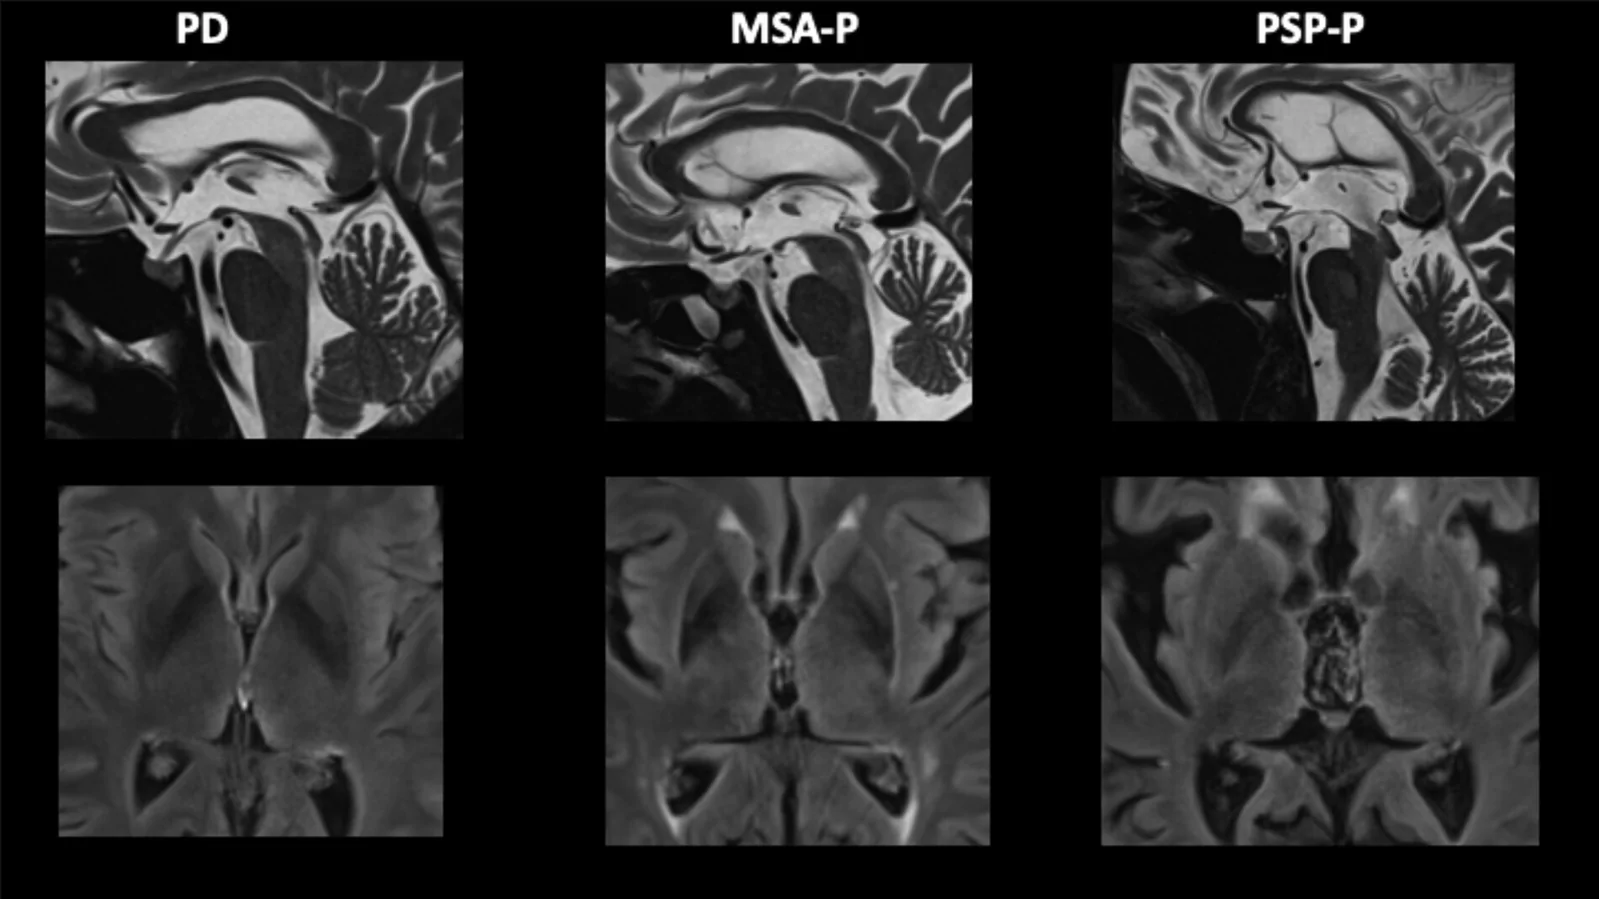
MRI features supporting the differential diagnosis of parkinsonian tremor syndromes. Conventional MRI is frequently normal in idiopathic Parkinson's disease (PD), whereas atypical parkinsonian syndromes may show characteristic structural abnormalities. In multiple system atrophy (MSA), these include putaminal atrophy and a hyperintense lateral putaminal rim in MSA-P, as well as pontocerebellar atrophy in patients with cerebellar involvement. Progressive supranuclear palsy (PSP) is characterized by midbrain atrophy, which may produce the sagittal “hummingbird” or “penguin” sign. MSA-P, multiple system atrophy parkinsonian type.
